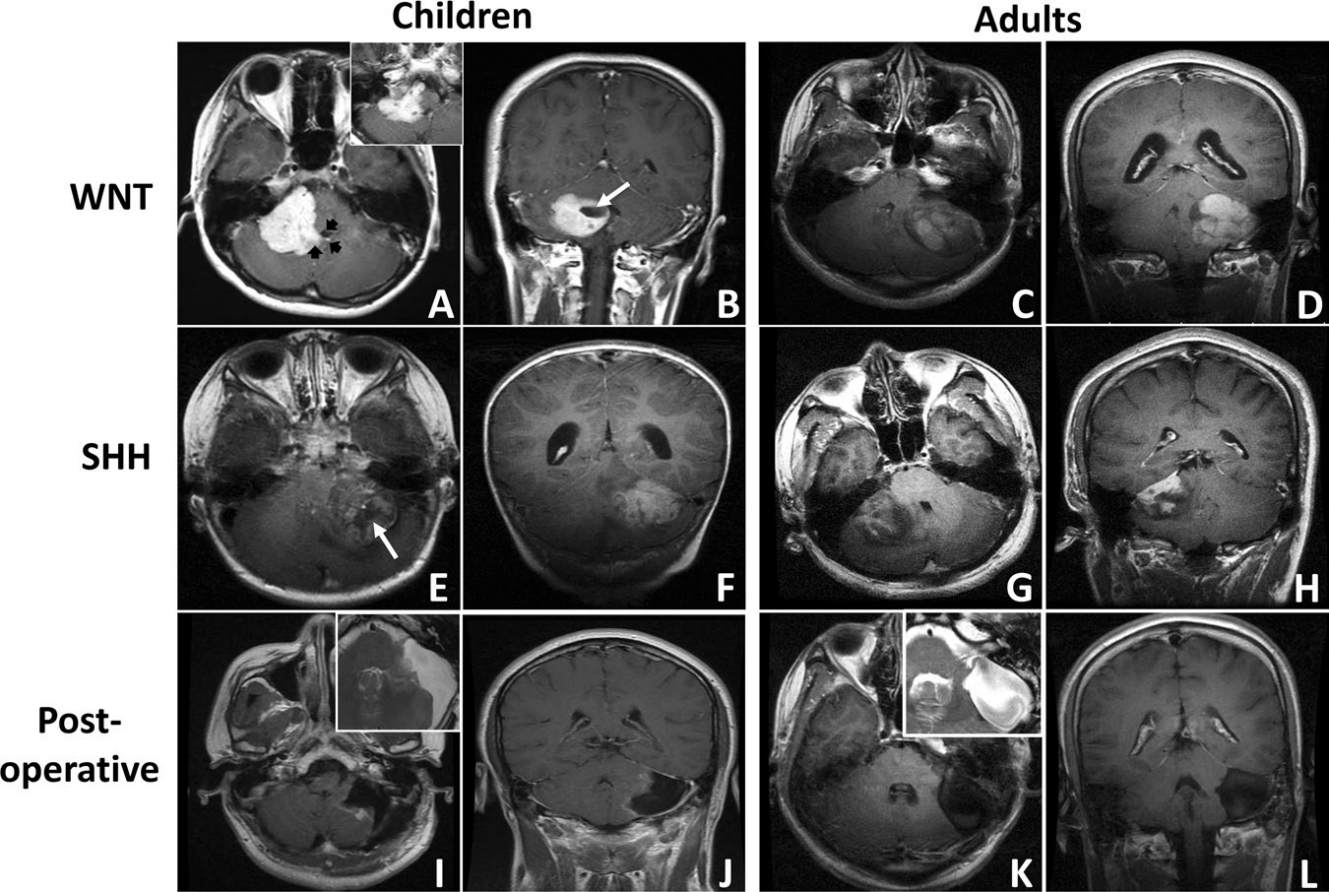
Exemplary magnetic resonance imaging (MRI) of cerebellopontine angle medulloblastoma (MB) (T1-weighted post-contrast axial and coronal MRIs). (A,B) A pediatric CPA WNT-MB with solid enhancement is shown (black arrow: the invasion of the 4^th^ ventricle is shown; white arrow: the focal cyst is shown; inset: prepontine cistern involvement). (C,D) An adult CPA WNT-MB with solid enhancement is shown (white arrow: the focal cyst is shown). (E,F) A pediatric CPA SHH-MB with heterogeneous enhancement is shown. (G,H) An adult CPA SHH-MB with heterogeneous enhancement is shown. (I,J) Postoperative images of a pediatric CPA SHH-MB are shown (inset: T2-weighted MRI; preoperative images: E,F). Damage to the left cerebellar hemisphere is shown. (K,L) Postoperative images of an adult CPA WNT-MB are shown (inset: T2-weighted MRI; preoperative images: C,D). Compression of the left cerebellar hemisphere without erosion is shown.
